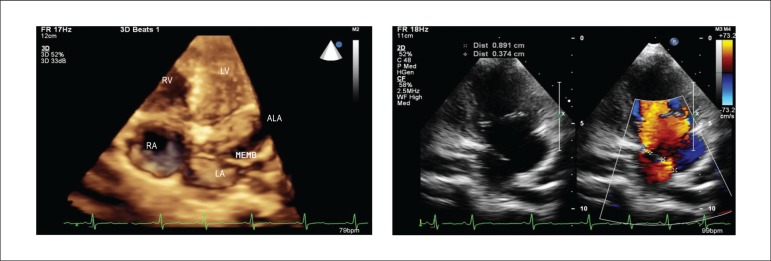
Echocardiograms in the 4-chamber apical plane in three-dimensional image
